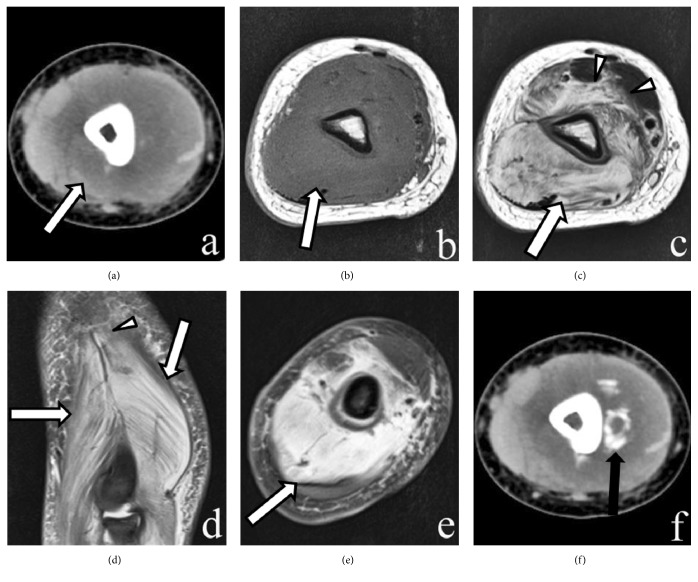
CT and MR imaging of the left forearm. (a) Axial plain CT image reveals an ovular, low-density, intramuscular mass-like lesion in the brachialis and triceps muscles. (b) Axial T1-weighted image shows an ill-defined isointense lesion. (c,d) Axial and coronal fat-suppressed T2-weighted image reveals a hyperintense lesion (large white arrow) with a “striate pattern” in the brachialis and triceps muscles with a pattern of edema in the brachialis and biceps muscles (arrowheads). (d) Enhanced fat-suppressed axial T1-weighted image shows that the lesion enhances intensely with a “striate pattern” in the muscle (large white arrow). Preservation of the muscle fascicles is noted in the lesion. (e) One month later, follow-up axial CT image shows a low-density lesion with a rim of well-defined focal and linear calcification (black arrow).
